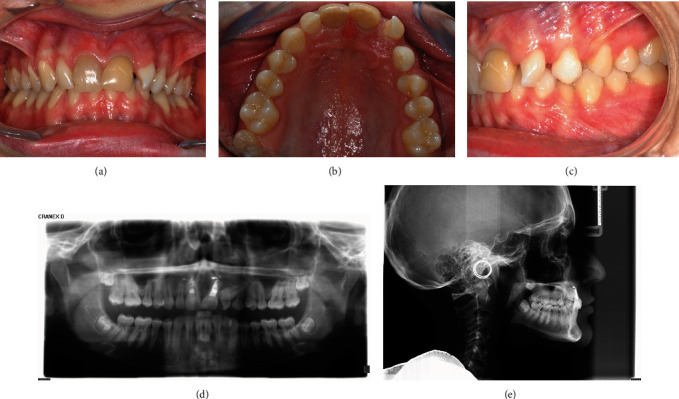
(a–c) Pretreatment intraoral and (d and e) radiographical recordings.
